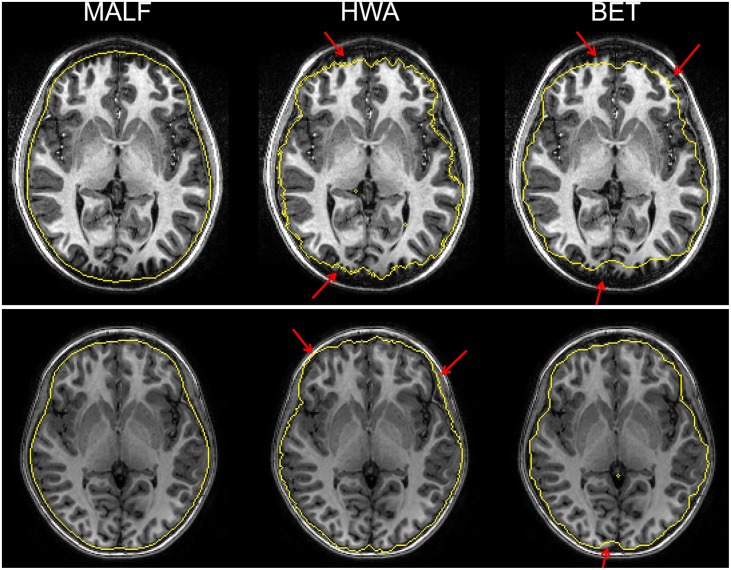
The top and bottom panels show automated skull-stripping results of individual subjects of the pediatric dataset, different for each panel, superimposed on their corresponding T1-weighted image. From left to right: results obtained from MALF, HWA, and BET. Regions pointed to with a red arrow indicate regions where inaccurate skull-stripping has occurred.
